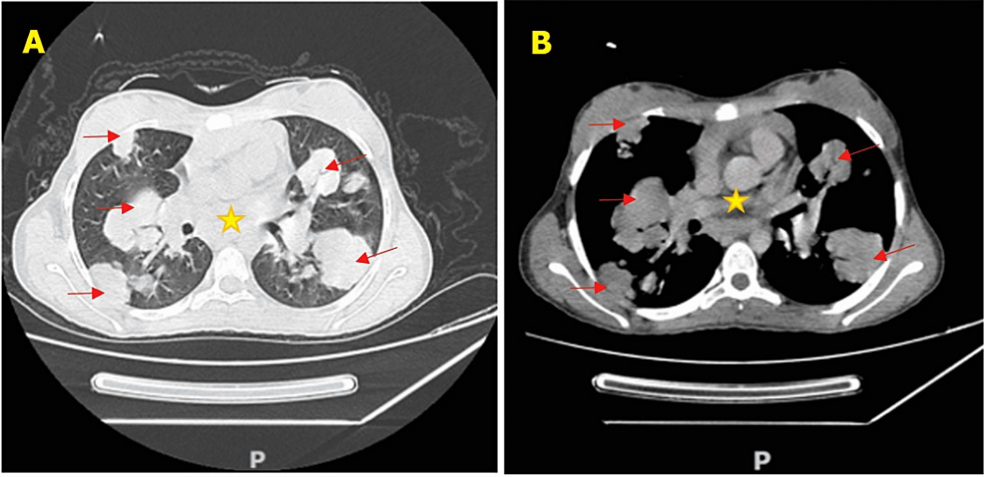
Axial chest CT scan: (A) lung window and (B) mediastinal window demonstrating bilateral and asymmetric pulmonary intra-parenchymal condensations (arrows) with mediastinal adenopathies (star).
